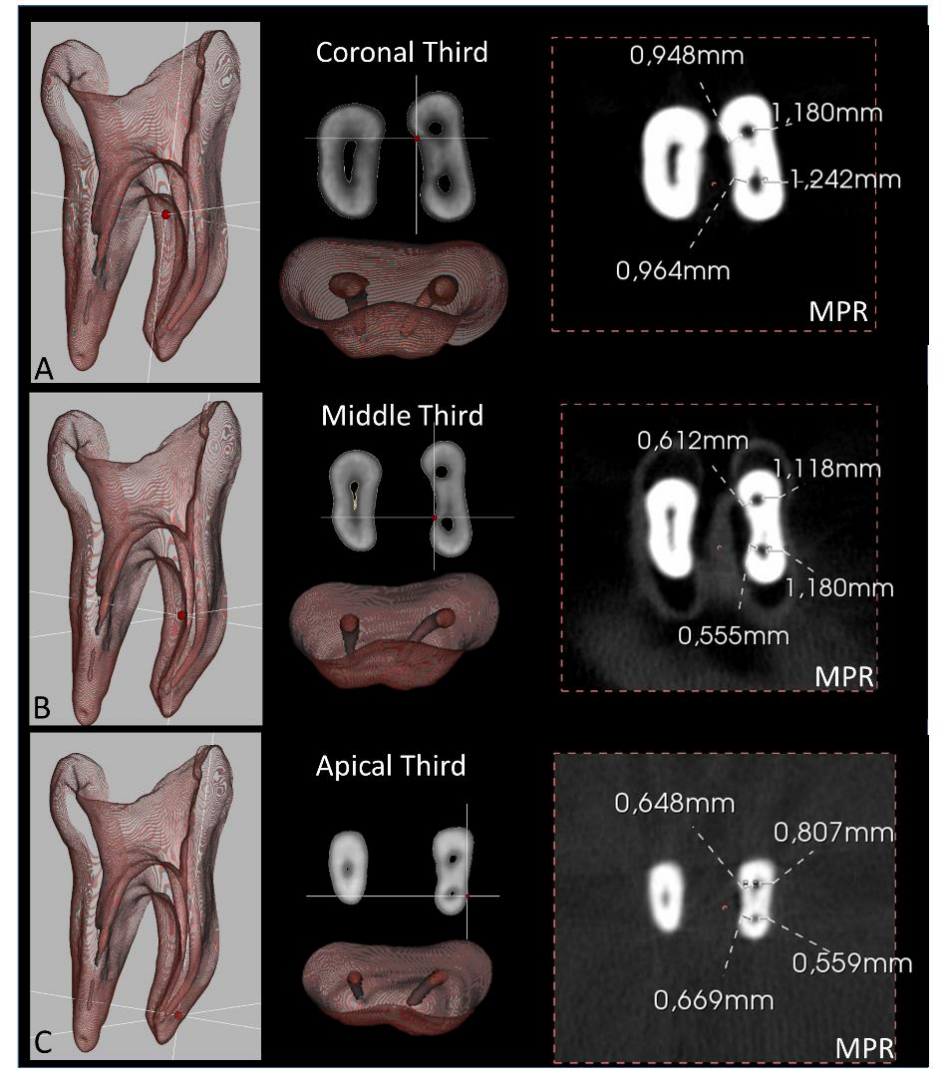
MPR in axial plane showing measurements and synchronized 3D volumetric reconstruction in coronal (A), middle (B), and apical thirds (C). A specific algorithm (pulp cavity) of CBCT e-Vol DX software was developed being able to suppress the cementum/dentin structures and show the relationship between the pulp cavity with the periodontal ligament, in addition, to viewing the volume, the final shape, and taper of the prepared root canal.
